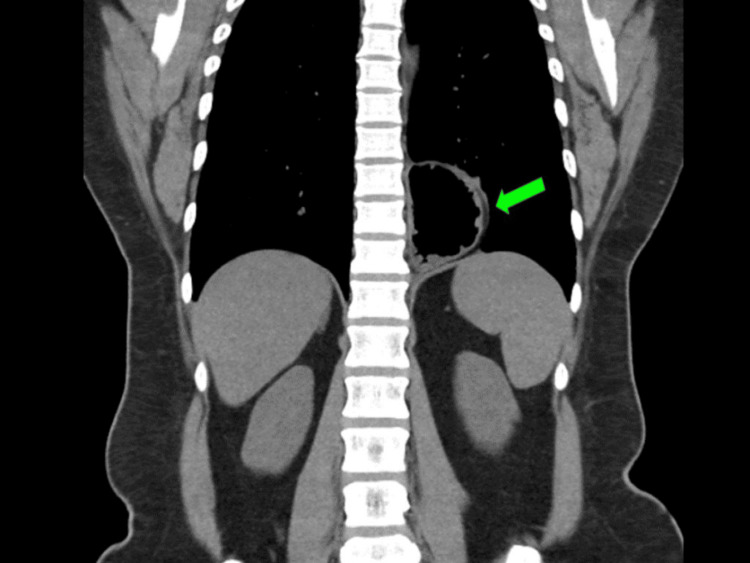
CT thorax and upper abdomen coronal view showing herniation of the stomach into the thoracic cavity (green arrow).
